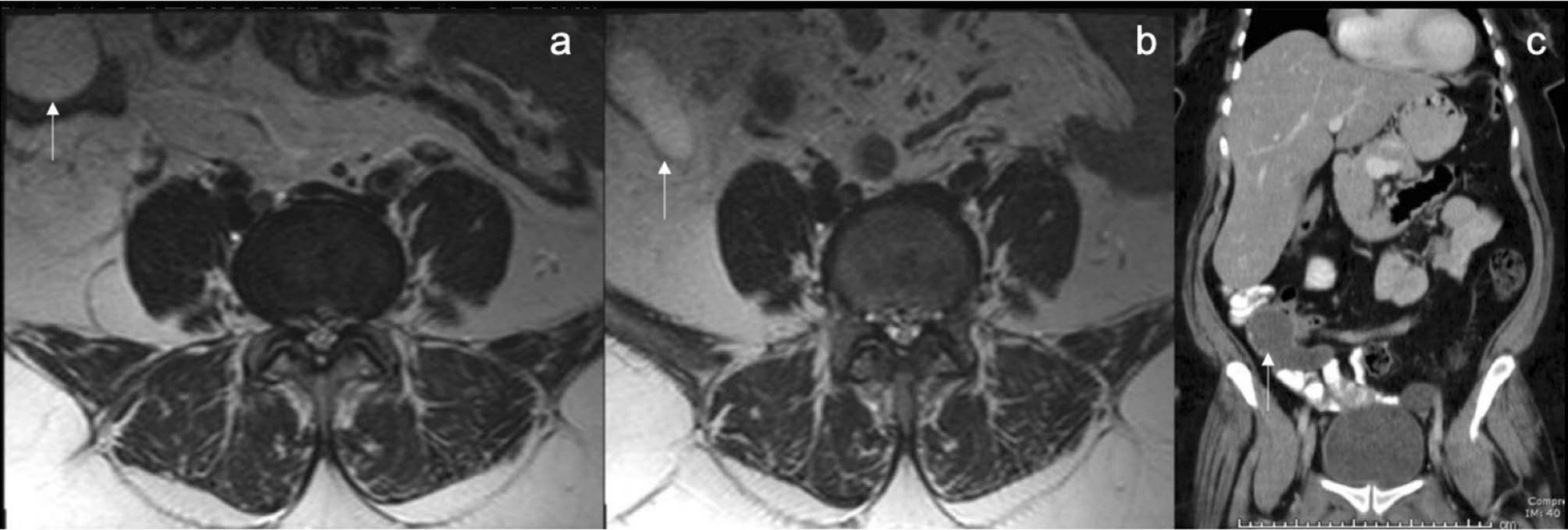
A 49-year-old female with appendiceal mucocele. (a) Axial T2 weighted image showing a cystic lesion in relation to the cecum (white arrow). (b) Axial T2 weighted image at a lower level showing the tubular shape of the cystic lesion (white arrow). (c) Coronal CT scan reconstruction with oral and IV contrast performed 2 years later demonstrated the same cystic lesion in relation to the cecum with faint wall calcifications (white arrow).
